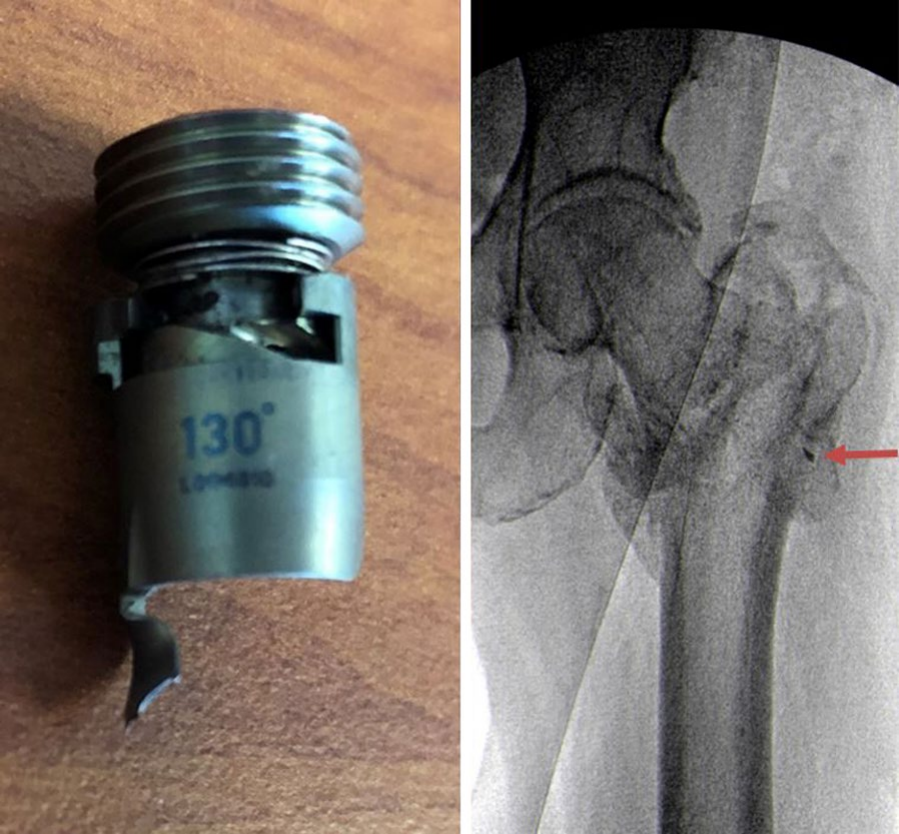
Visualized on the left is the characteristic deformity and fracture of the TFNa set screw that occurs if the lock prong is damaged during implant extraction. Failure to fully elevate the set screw prior to helical blade removal will cause the lock prong to deform and fracture more distally toward its tip. The fractured lock prong in this case remained within the patient as a retained foreign body visible on X-ray marked by the arrow in the image to the right
